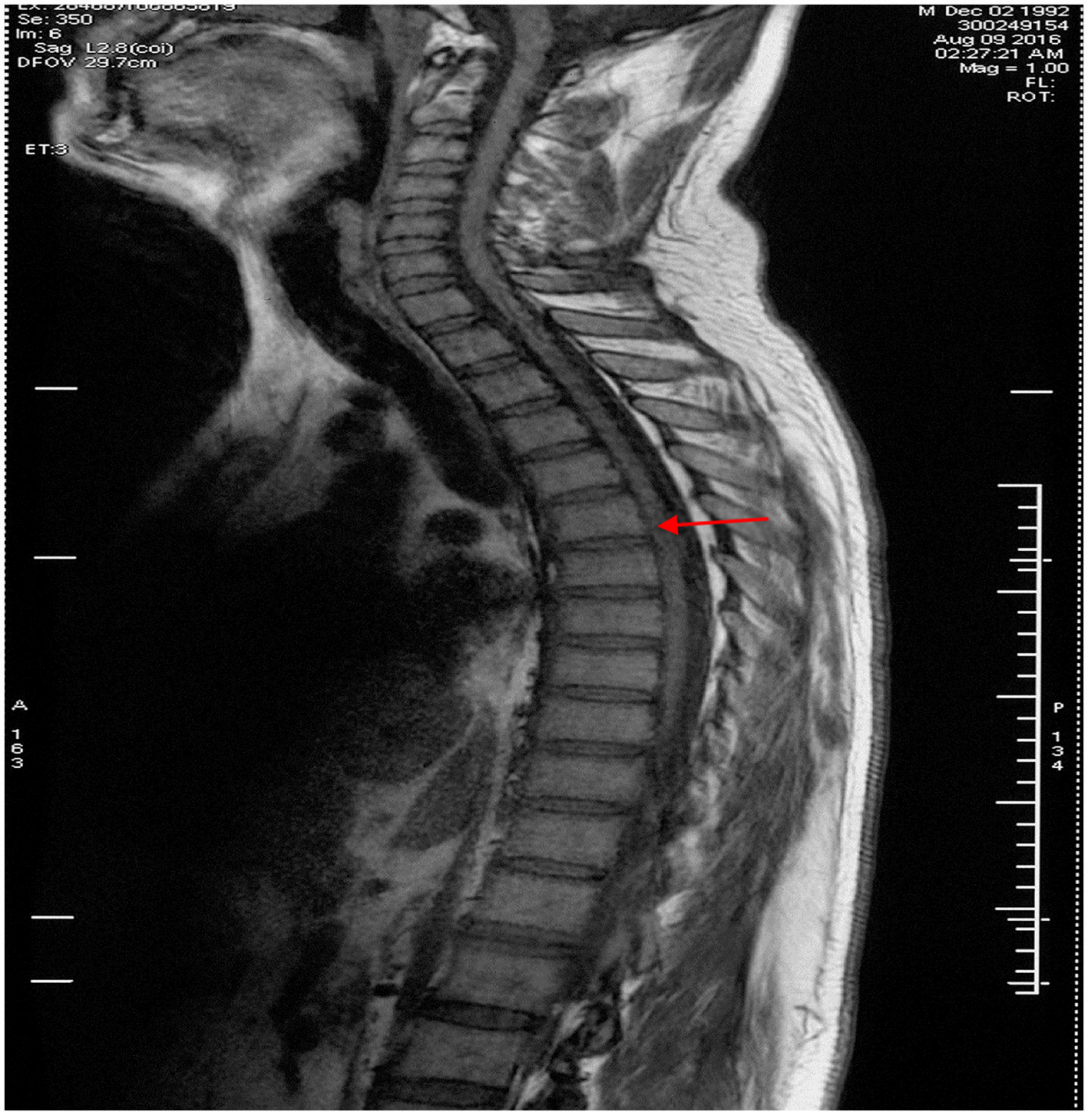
Thoracic spinal MRI image in the proband. Thoracic spinal MRI revealed thinning of the thoracic spinal cord with volume loss but no abnormal signal in the cord on sagittal T1-weighted imaging, as indicated by the red arrow.
